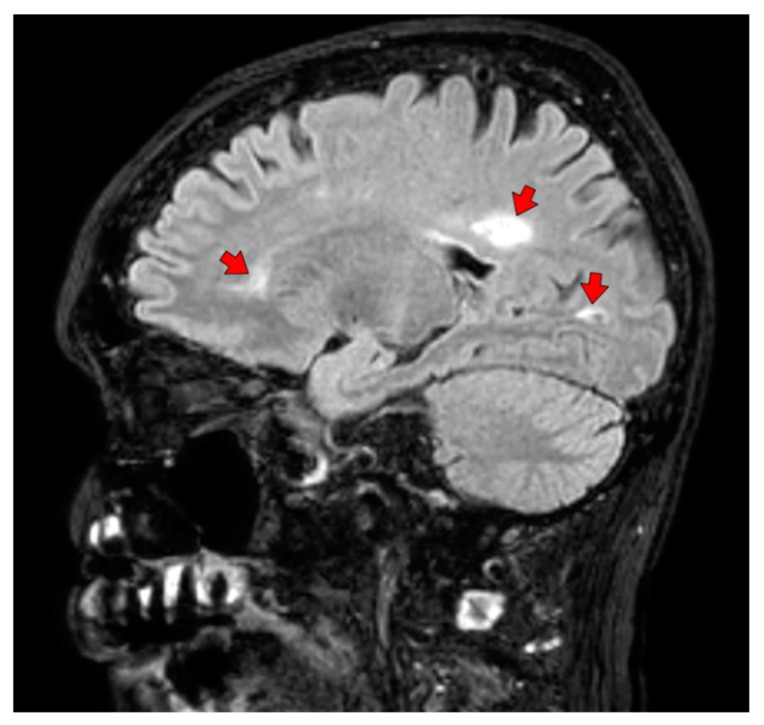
A fluid attenuation inversion recovery (FLAIR) image from a patient with sarcoidosis showing silent white matter hyperintensities.
